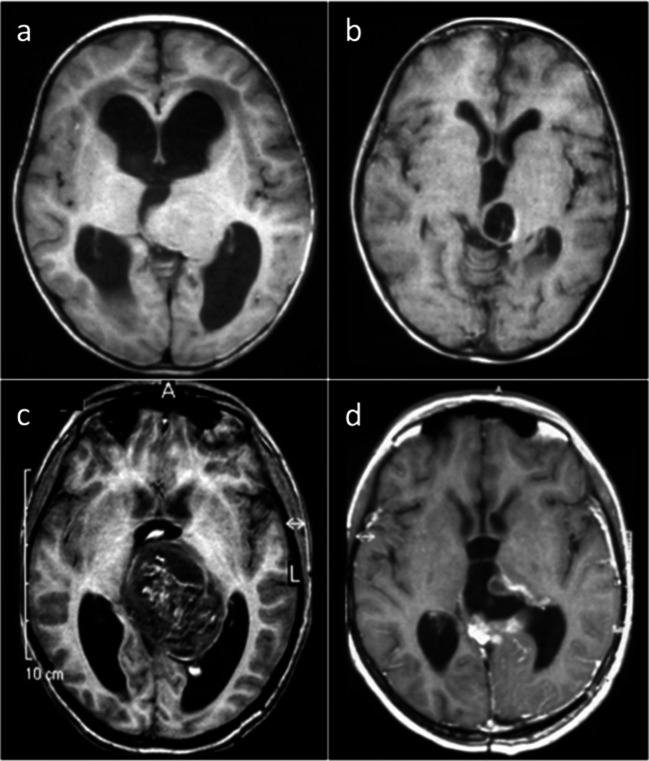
Case1 (a) axial T1-weighted MRI depicting a left thalamic tumor, (b) stable tumor remnant 5 years after STR via a suboccipital-transtentorial approach, (c) 9 years after diagnosis rapid progression as GBM with intratumoral bleeding, (d) early postoperative MRI after a combined occipital-transcortical and suboccipital-transtentorial STR, the patient rapidly progressed despite chemotherapy/radiotherapy
